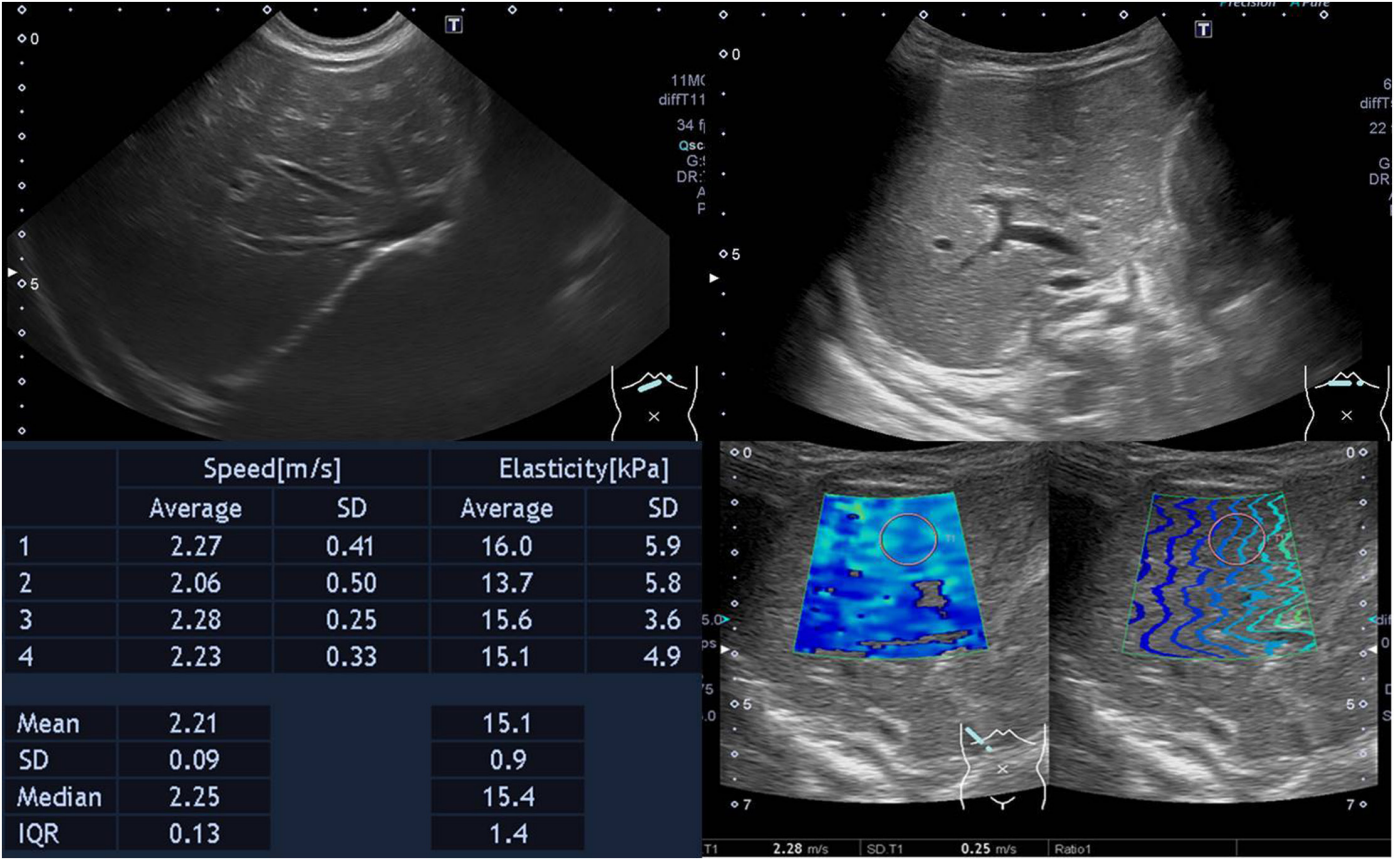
Abdominal sonography at 2 months old with increased shear wave elastography (Toshiba Aplio 500).
